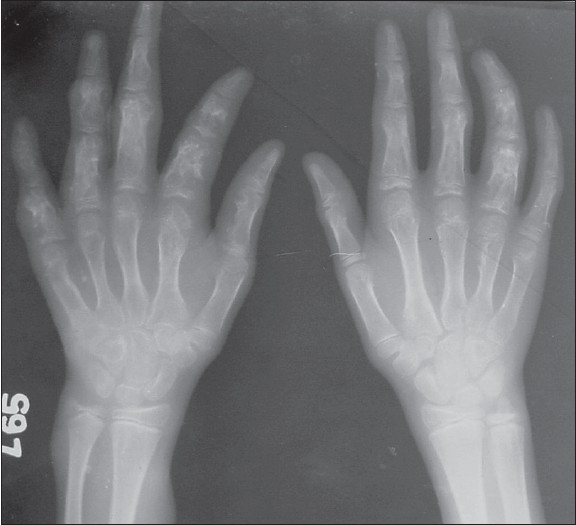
Radiograph showing radiolucent expansile lesions involving the metacarpals and phalanges bilaterally.
